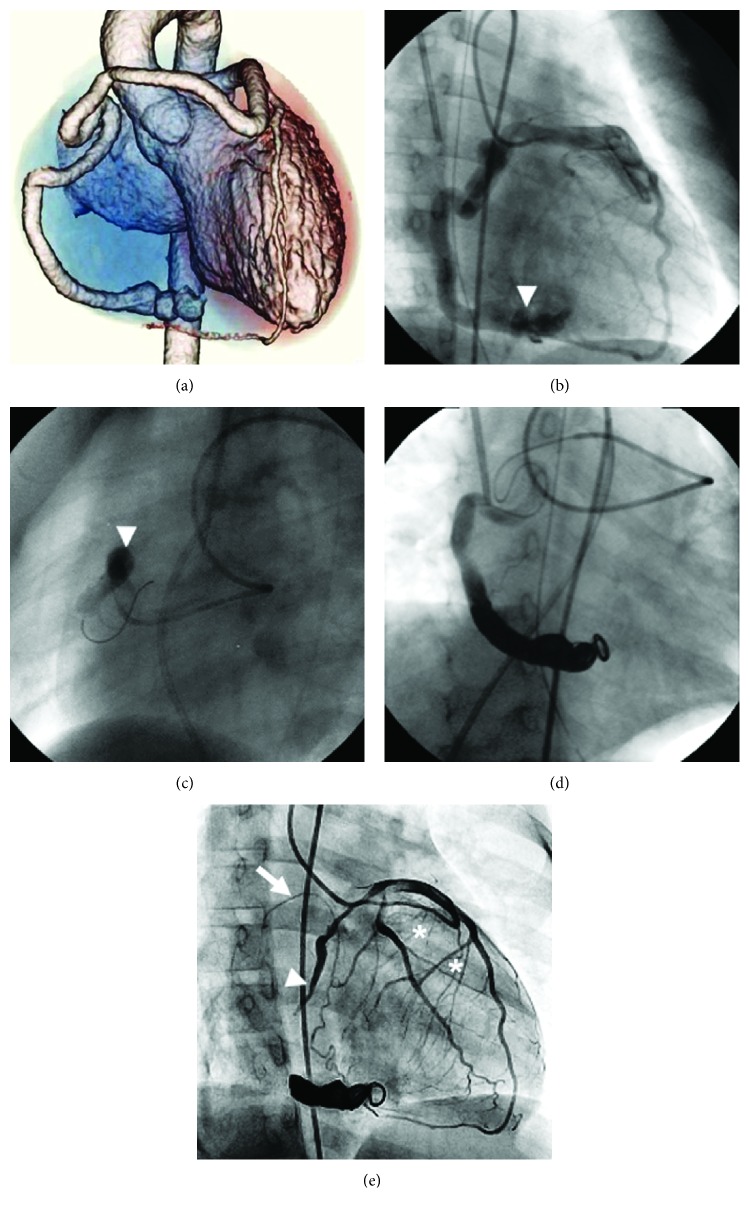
(a) 320-row coronary CTA. The single coronary artery view shows the anterior course of the separate right coronary artery coming off the LAD. This is the anterior view. (b) Selective coronary angiography of the LMT shows the LAD and the LCX with weak contrast effects and a CAF with “multiple caliber change” of the RCA to the right ventricle (arrowhead). (c) Occlusion test on the proximal site of CAF using an occlusion balloon (arrowhead). (d) Engaged microcatheter in the distal RCA and embolization using a detachable coil. (e) One year after coil embolization, selective LMT coronary angiography clearly distinguishes the contrast effect of the LAD and LCX, the sinus node branch branching from LCX (white arrow), and right ventricular branches from the proximal side of the RCA (asterisks). The proximal end of the coil-embolized CAF formed a thrombus and occluded (arrowhead). CAF: coronary artery fistula; CTA: computed tomography angiography; LAD: left anterior descending coronary artery; LCX: left circumflex coronary artery; LMT: left main coronary trunk artery; RCA: right coronary artery.
